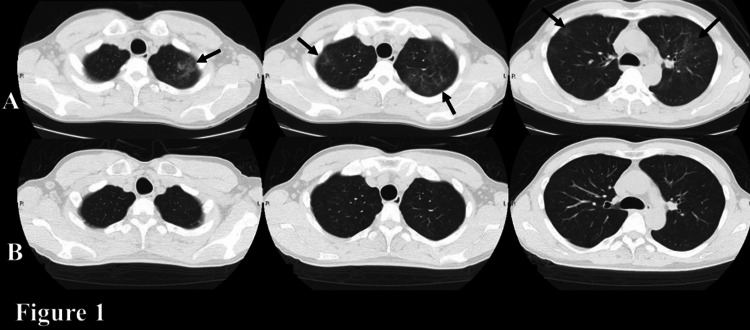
Section of axial chest CT. (A) Ten days prior to admission (in the figure above). (B) On admission (in the figure below). Chest CT revealed diffuse ground-glass opacity (GGO) (arrows) in (A) and improvement of GGO in (B).
